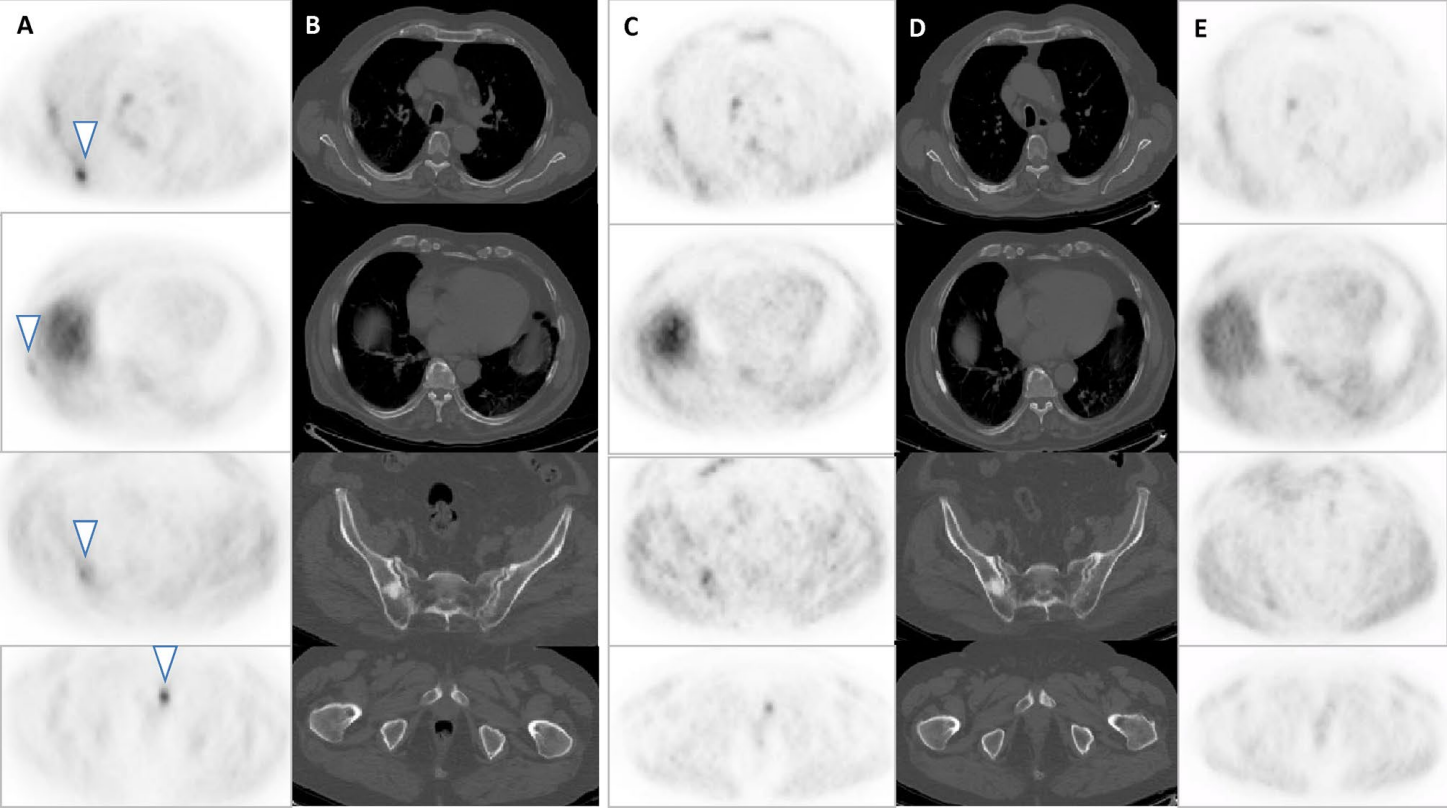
Patient ≠ 2. Axial slices of baseline FCH PET/CT (A, B) showing four lesions with uptake and blastic reaction (arrow-heads). Axial slices of interim FCH PET/CT (C, D) show stability in lesion number, with reduction of metabolism. Axial slices of end-treatment FCH PET portion (E) show a reduction of uptake with minimal visual detection of lesions.
